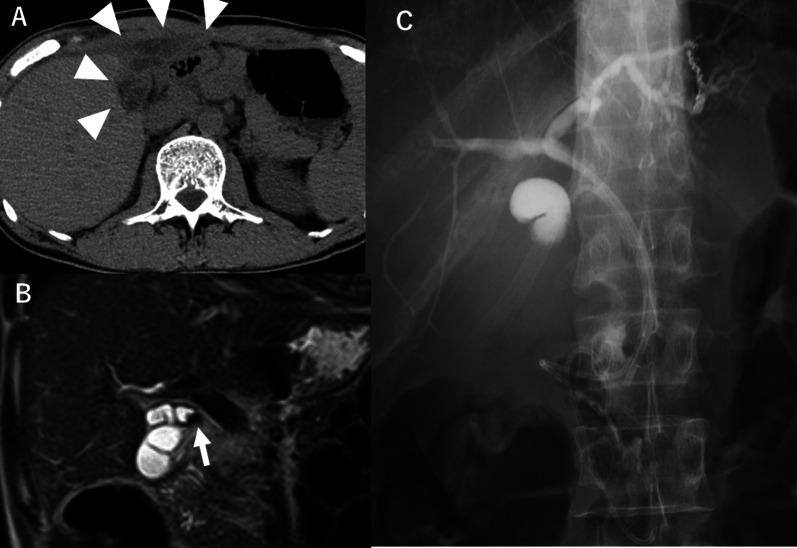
Case 2. a Plain abdominal computed tomography showed remnant gallbladder (47 × 29 × 28 mm) with intraabdominal abscess under the abdominal wall (arrow head). b A small stone was found in the cystic duct (arrow). c The EBS and EGBD tubes were placed in the common bile duct and the remnant gallbladder by ERCP, respectively
